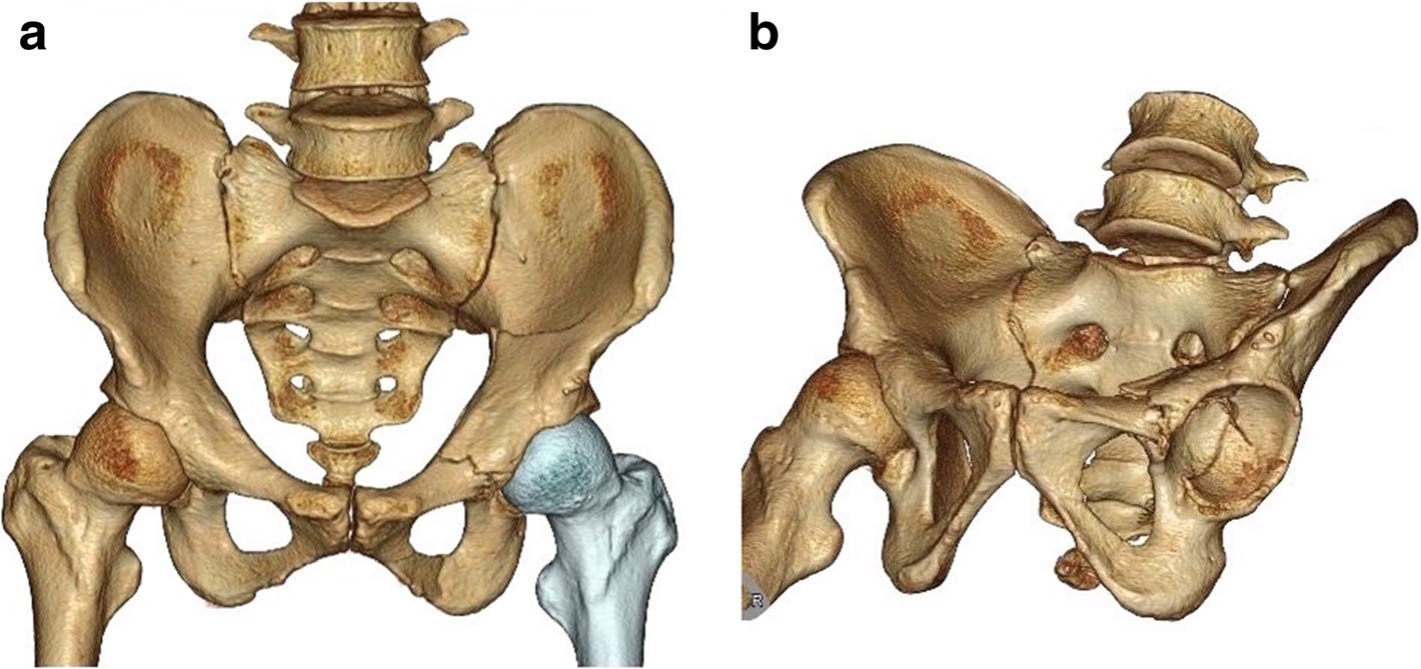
Preoperative 3D-CT. a Frontal view. b Left oblique view. A fracture of the left innominate bone and a fracture of the left acetabulum are observed
